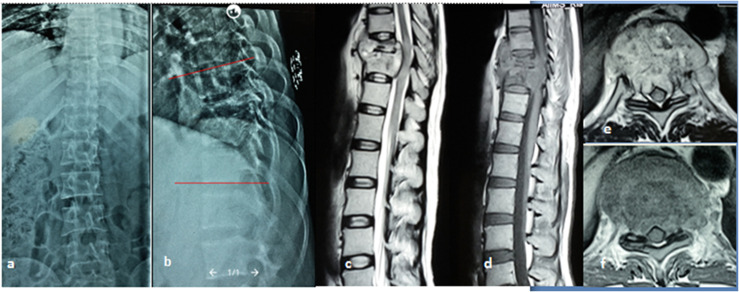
A 42-year-old female diagnosed as case of Potts spine D9–D10 with neurological deficit. (a) X-ray AP and (b) lateral view showing fuzziness of end plates of D9–D10 with kyphosis of 16° measured using cobs method. (c) MRI sagittal T2WI, (d) sagittal T1WI, (e) axial T2WI, (f) axial T1WI showing paradiscal involvement of D9–D10 vertebral bodies with endplate destruction and altered marrow signal intensity, prevertebral and epidural collection causing loss of anterior CSF around cord and cord compression.
